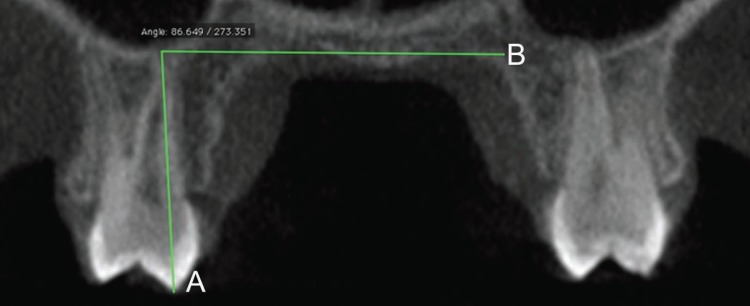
Angle measured between a line from the palatal cusp tip to apex of the anchor tooth (A) and a horizontal line the at the level of hard palate (B). Legend: This measurement expressed to the angle of anchor tooth quantified through ST-CBCT in each patient before and after rapid maxillary expansion.
